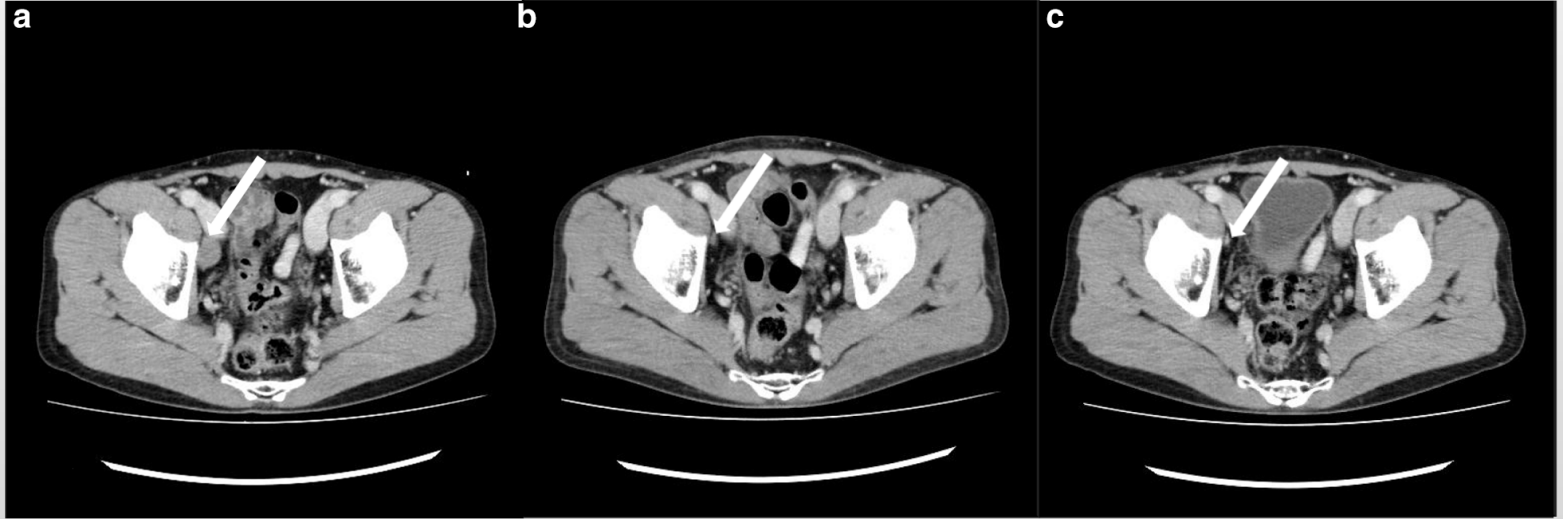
Contrast enhanced pelvic CT images before chemotherapy. a Enlarged right external iliac lymph node (white arrow). b Clinical complete response was confirmed after chemotherapy (white arrow). c Clinical complete response continued 12 months after chemotherapy (white arrow)
